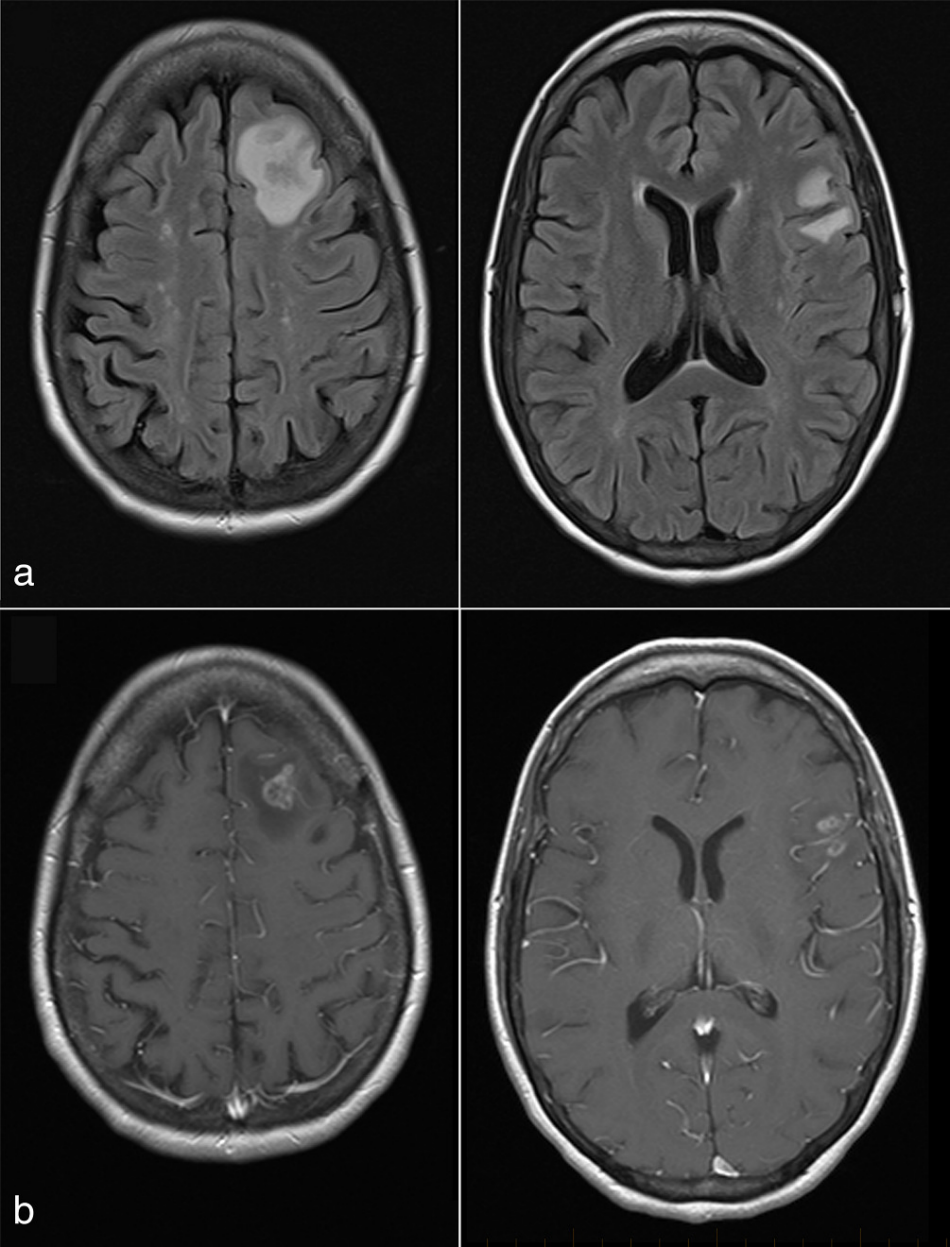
(a) Fluid-attenuated inversion-recovery and (b) *T*_1 _weighted contrast-enhanced MRI showing a 1.4-cm irregular ring-like enhancing lesion within the superior left frontal lobe at the grey–white junction (*T*_1_ and *T*_2_ isointense with surrounding vasogenic oedema) and a 1.3-cm lesion with a similar appearance in the region of the frontal operculum, also at the grey–white interface.
